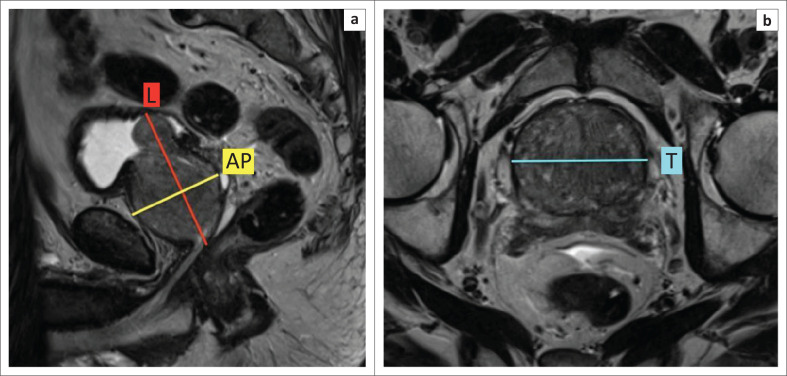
Recommended measurements for calculation of prostate volume at magnetic resonance imaging. Maximum longitudinal (L) diameter and maximum antero-posterior (AP) diameter measured on mid-sagittal T2-weighted imaging (T2WI) (a) and maximum transverse (T) diameter measured on axial T2WI (b).
